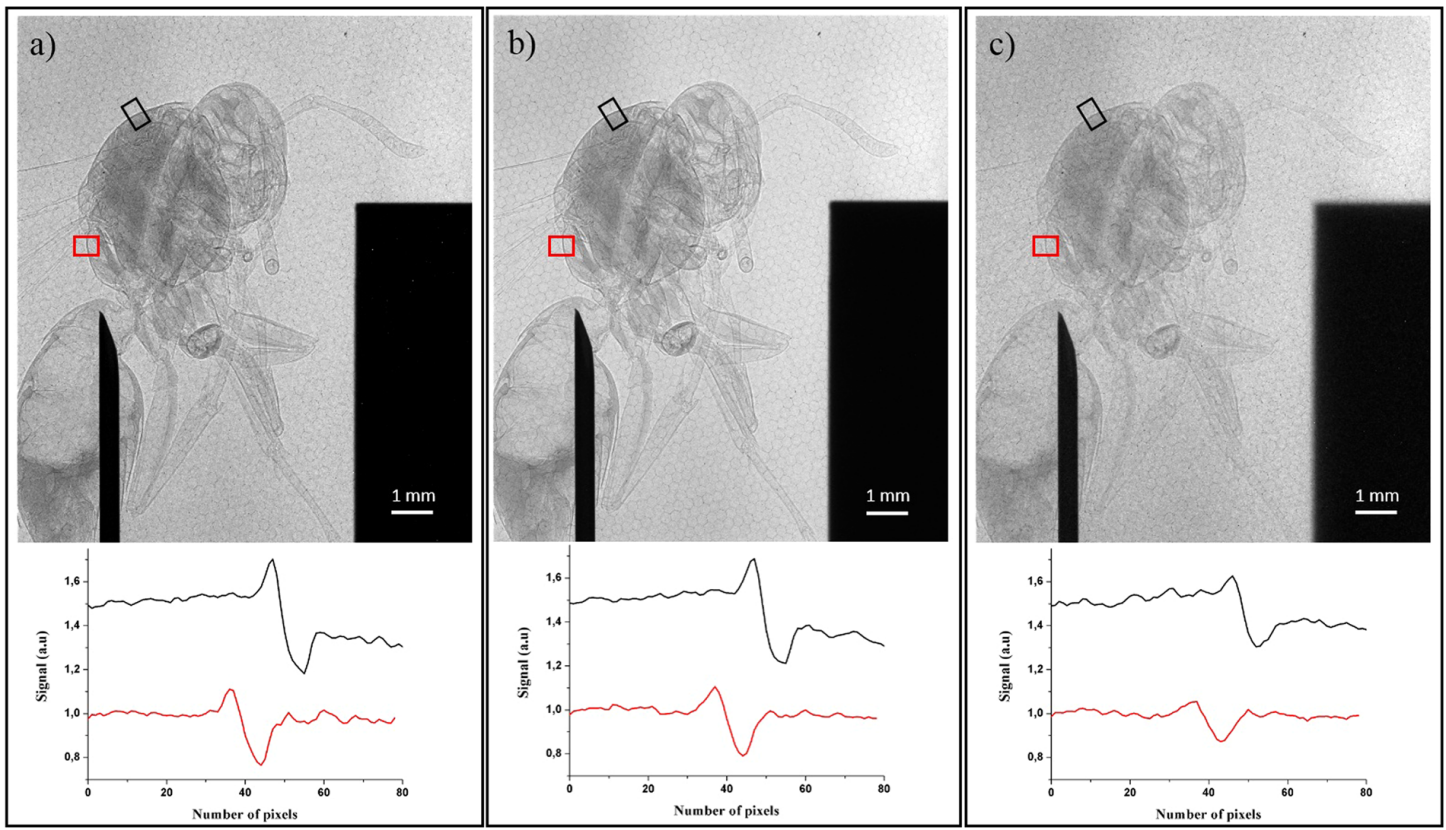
Raw x-ray phase contrast images of the wasp. The knife edge is simultaneously imaged to control the corresponding effective x-ray source size. Below each image are plotted two line profiles of the signal perpendicular to the edge for 2 thorax zones (black and red rectangles). (a) for I = 2.7 × 10^17^ W/cm^2^, (b) for I = 1.0 × 10^18^ W/cm^2^, (c) for I = 7.2 × 10^18^ W/cm^2^. The honeycomb structure in the background is related to the optical fibers which redirect the converted x-ray signal to the CCD array of the detector (see *Methods*).
